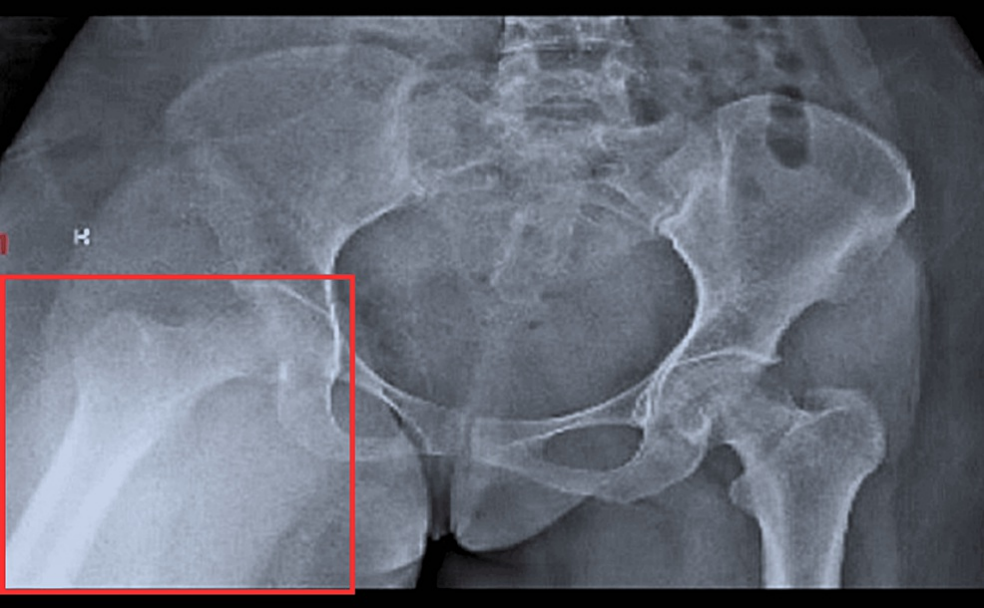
Pre-operative X-ray of right-sided hip The red square shows a fixed flexion deformity of 50^o^.
